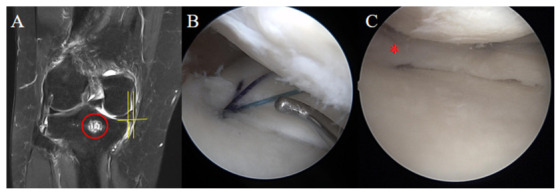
Representative Case of MMPRT Repair and Second-Look Arthroscopy. (A) Preoperative coronal T2-weighted MRI of a 55-year-old woman with a painful popping event, showing medial meniscus posterior root tear with medial meniscus extrusion (3.52 mm). A red circle indicates the subcortical bone cyst at the tibial attachment site. Yellow lines demonstrate the method used to measure medial meniscus extrusion. (B) Arthroscopic view during transtibial pullout repair. According to the tibial tunnel position, the torn root was repaired using a simple vertical suture configuration. (C) Second-look arthroscopy performed 6 months postoperatively. The red asterisk (*) indicates the repaired medial meniscus posterior root, which demonstrated partial healing with maintained continuity but increased laxity on probing. MMPRT: medial meniscus posterior root tear.
